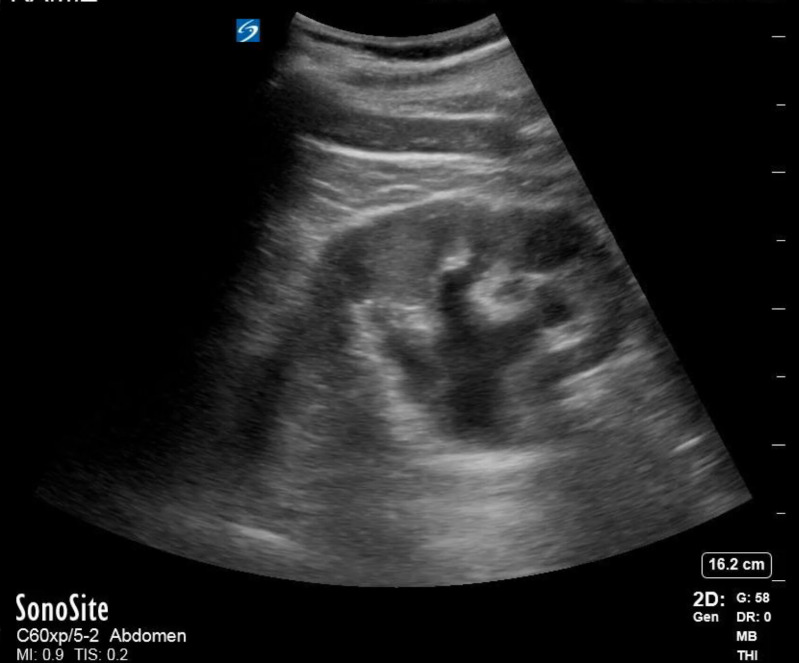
Renal point of care ultrasound (POCUS) demonstrating moderate left-sided hydronephrosis without surrounding free fluid.
